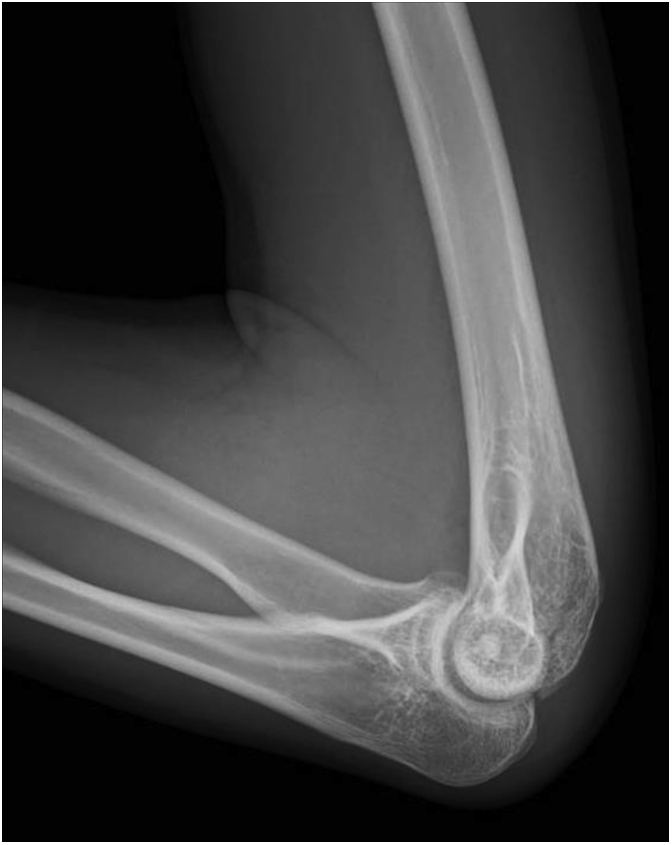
Plain radiograph of the elbow joint after reduction. Lateral view. An anatomical reduction was obtained.
